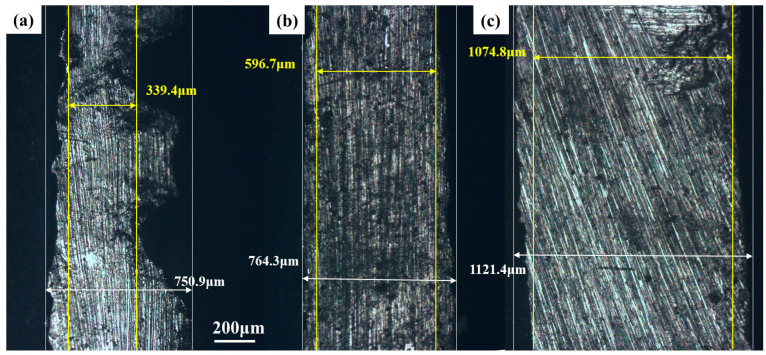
Thicknesses of the tin sheets after ultrasonic treatment (a); after conventional treatment (b); raw material tin sheet (c).
